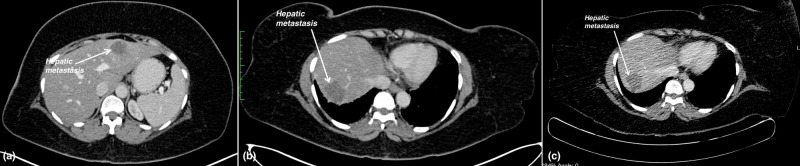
(a) PET-CT shows hepatic metastasis in the left lobe of the liver. (b) PET-CT shows hepatic metastasis in the right lobe of the liver. (c) PET-CT shows stable hepatic metastasis in the right lobe of the liver. PET-CT, positron emission tomography-computed tomography.
